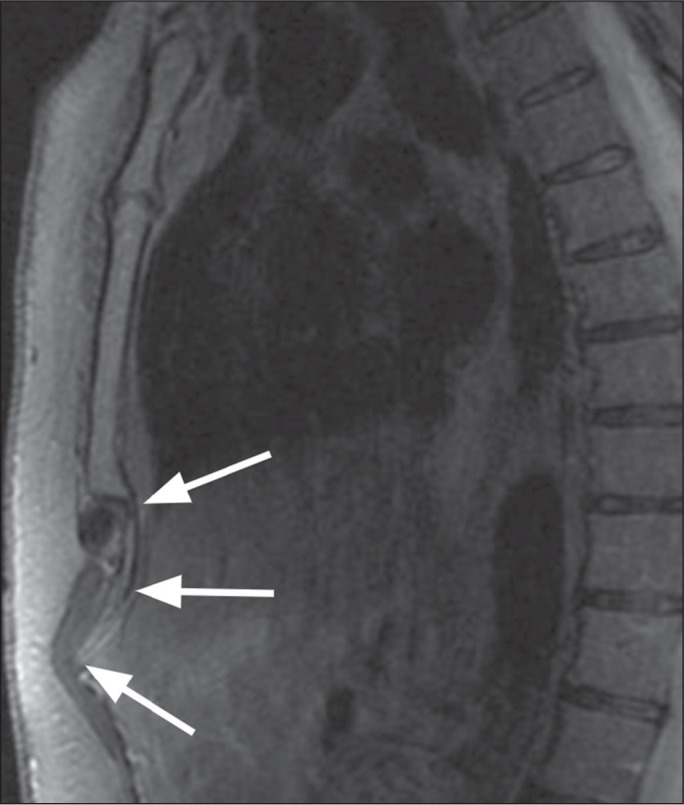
Anterior curvature of the xiphoid process. Sagittal T1-weighted MRI
